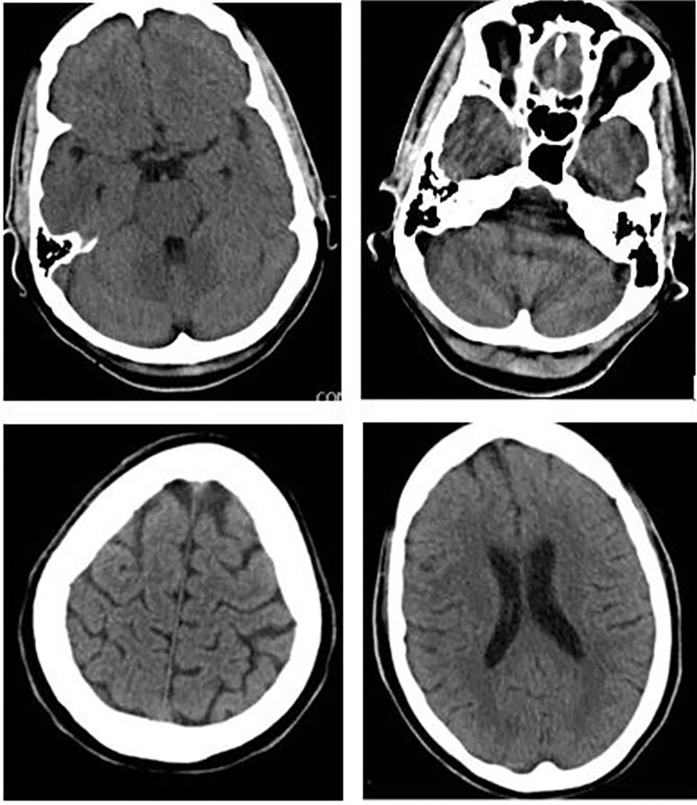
Representative images from the patient’s computed tomography of the head, showing no acute disease.
